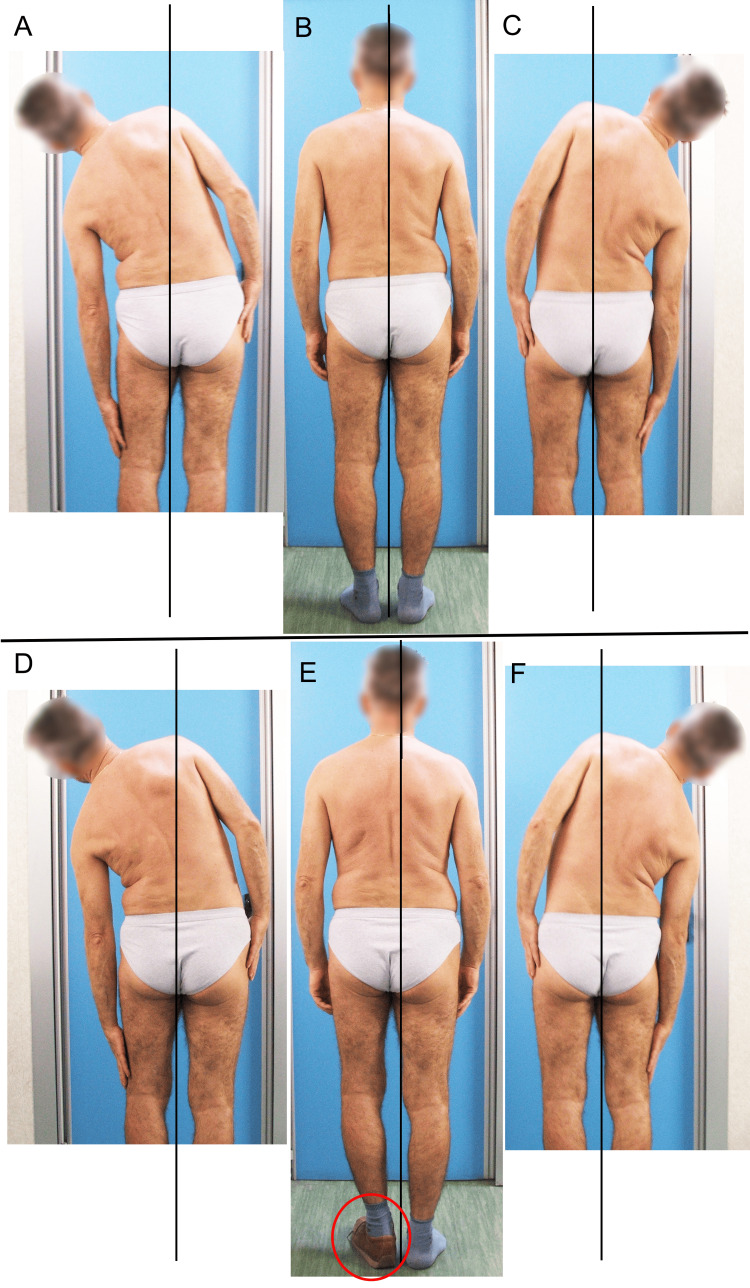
This subject acquired LLI after bone maturity due to a femoral fracture. A patient with the left limb (on X-rays appears to be approximately 23 mm) shorter than the right. In the top row of images, the photograph on the left (A) features the patient demonstrating maximal left flexion; in the center (B), the neutral upright posture; and on the right (C), maximal right flexion. Less lateral flexion on the side of the longer limb is clinically evident. The bottom row of images (D, E, F) presents the same poses by the same subject wearing on the left foot a shoe with a heel of approximately 20 mm. A comparison of the two scenarios indicates that wearing the shoe allows a greater symmetry of the lateral flexion between the two sides. Image Credit: Author Saverio Colonna
